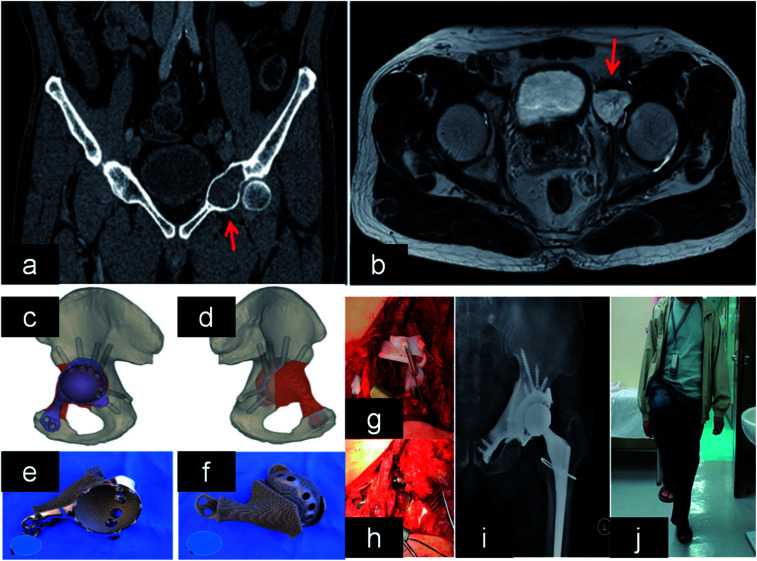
(a and b) Lesion and its boundary located in the MRI and CT. (c and d) The 3D pelvic model showed the implant reconstruction and screws fixation. (e) The outer view showed the acetabular cup with screws holes for fixation. (f) The back side showed the porous structure that was in contact to bone. (g and h) After tumor resection, the implant was fitted to the bone defect perfectly and stabilized with screws. (i and j) X-ray showed good implant alignment, and patient could stand with single leg at 10 months after the surgery. This figure has been reproduced from ref. 167 with permission from Taylor & Francis.
